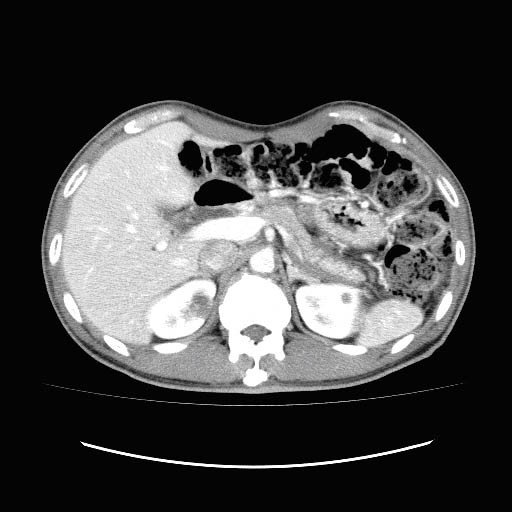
CT showed poorly enhanced mass lesions (left, 10 × 10 mm; right, 18 × 14 mm) in the upper part of both kidneys.
